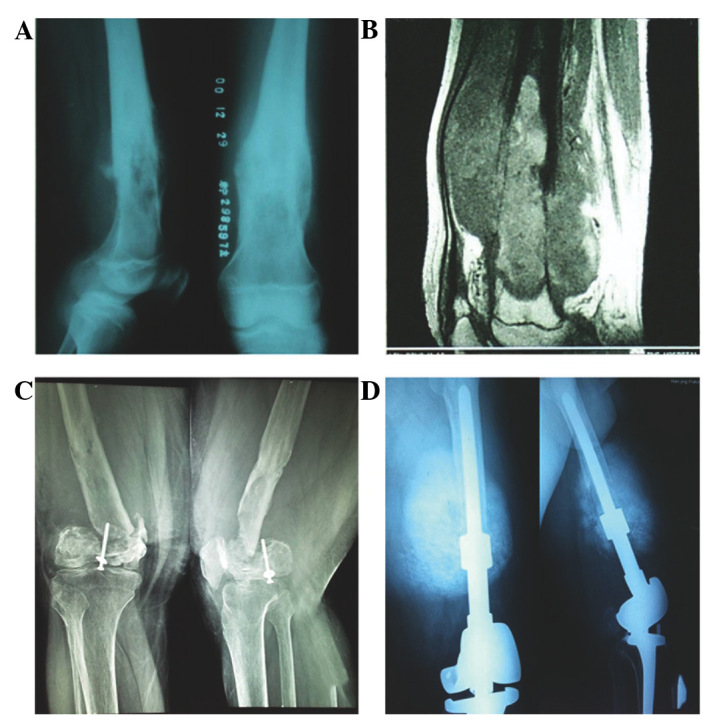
A male patient with late recurrent osteosarcoma 10 years post-surgery. (A) X-ray imaging showing osteoblastic destruction of the left distal femur, partial osteolytic changes, a visible periosteal reaction and a soft tissue mass. (B) MRI showing a mixed high and low signal intensity in the left distal femur, which formed a large soft tissue mass. (C) X-ray showing the allografting bone resorption and femoral condyle fragmentation at 8 years post-surgery. (D) X-ray imaging revealing a tumor shadow between the prosthesis and the host bone in the middle of the right femur with a soft tissue mass at 9 months after the second surgery. The lesion was confirmed to be an osteosarcoma by a post-operative pathological examination.
